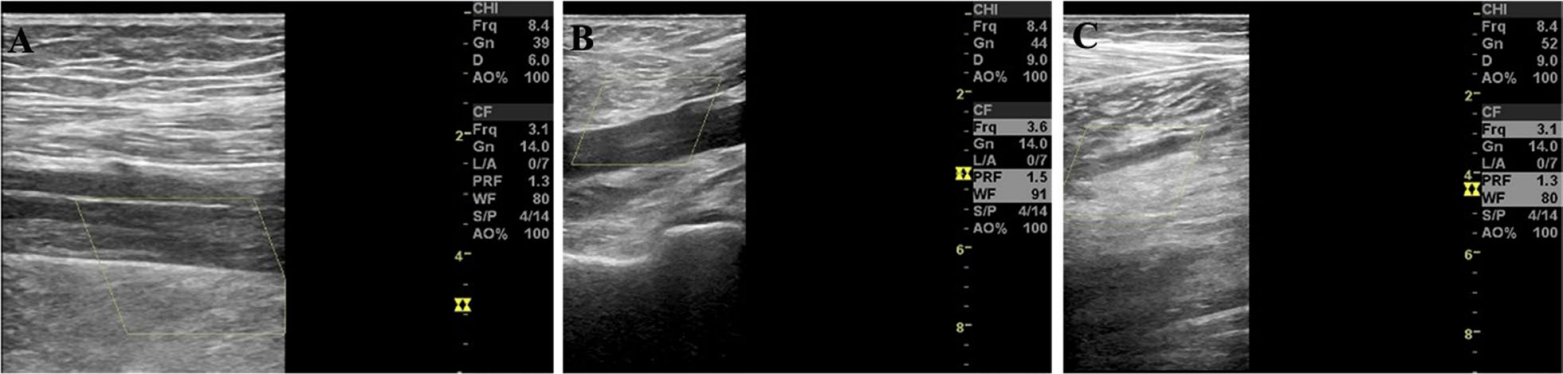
Color Doppler flow images revealing DVT involving the lower extremities. A DVT in the superficial femoral vein of the right leg. B DVT in the popliteal vein of the right leg. C DVT in the intermuscular vein of the right leg. No signals of blood flow were shown above. DVT, deep venous thrombosis
